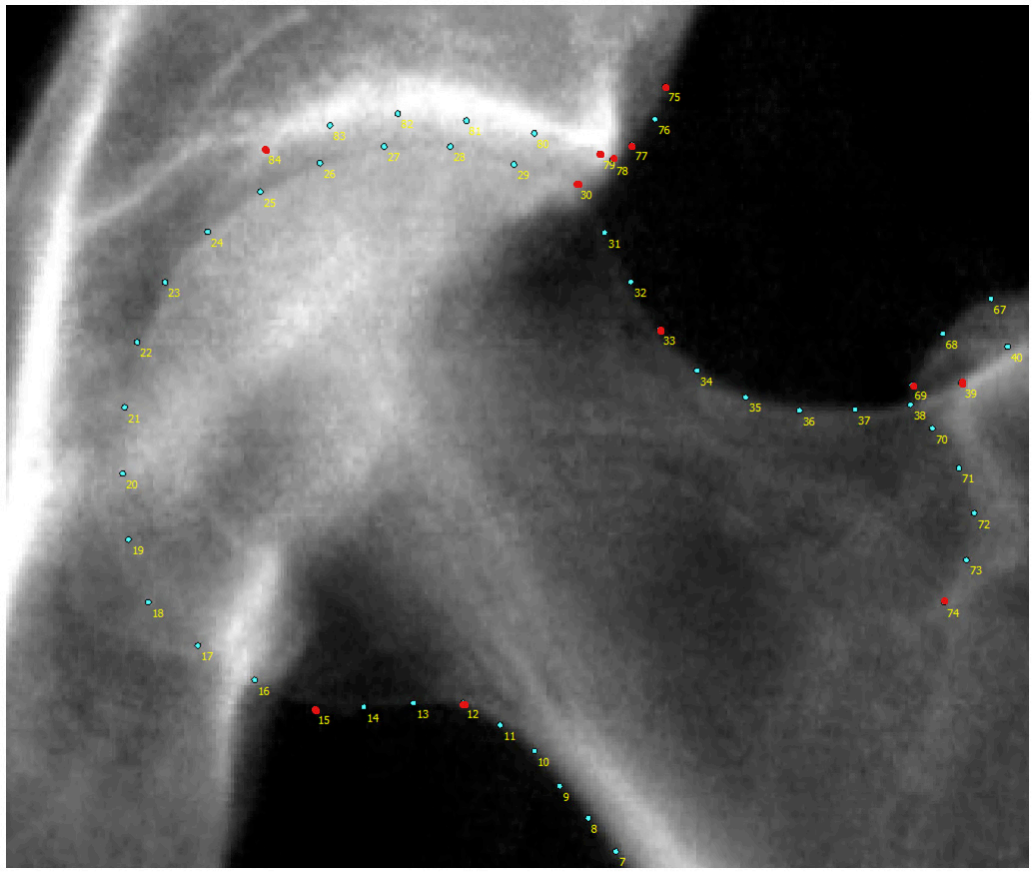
A representative dual-energy x-ray absorptiometry (DXA) scan from UK Biobank (UKB) with all femoral head and acetabular outline points labelled. The points marked in red are key anatomical landmarks. Only points 8-39 were used in this study. The acetabular points and the remaining proximal femur points were not necessary.
